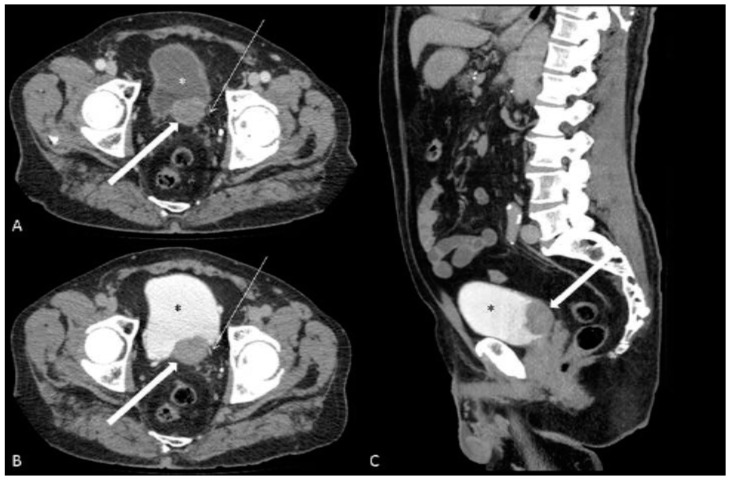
(A–C) CT urography of neuroendocrine carcinoma of the urinary bladder in an 83-year-old man presenting for haematuria. Axial portal venous phase (A), axial (B) and sagittal (C) excretory phase, demonstrate the presence of a lesion (thick arrow) of the posterior wall and trigonal region of the bladder (*). Please note the ring enhancement and the absence of necrosis and calcification. The left ureter (thin arrow) runs immediately lateral to the lesion; its appearance is unremarkable.
